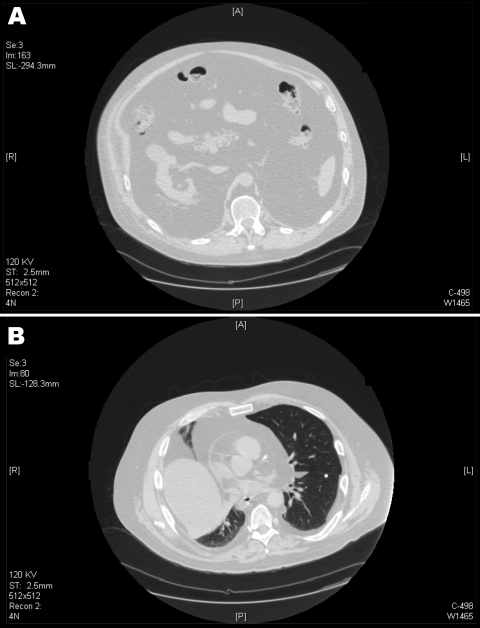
Computed tomography scans of the patient, showing marked visceral adipose tissue in the abdomen (A) and thorax (B). Diffuse intrabadominal, retroperitoneal lipomatosis, and herniation of the mediastinum can be seen through the esophageal hiatus. Intrapericardial adipose infiltration and adipose tissue bilaterally are seen within the pleura.
